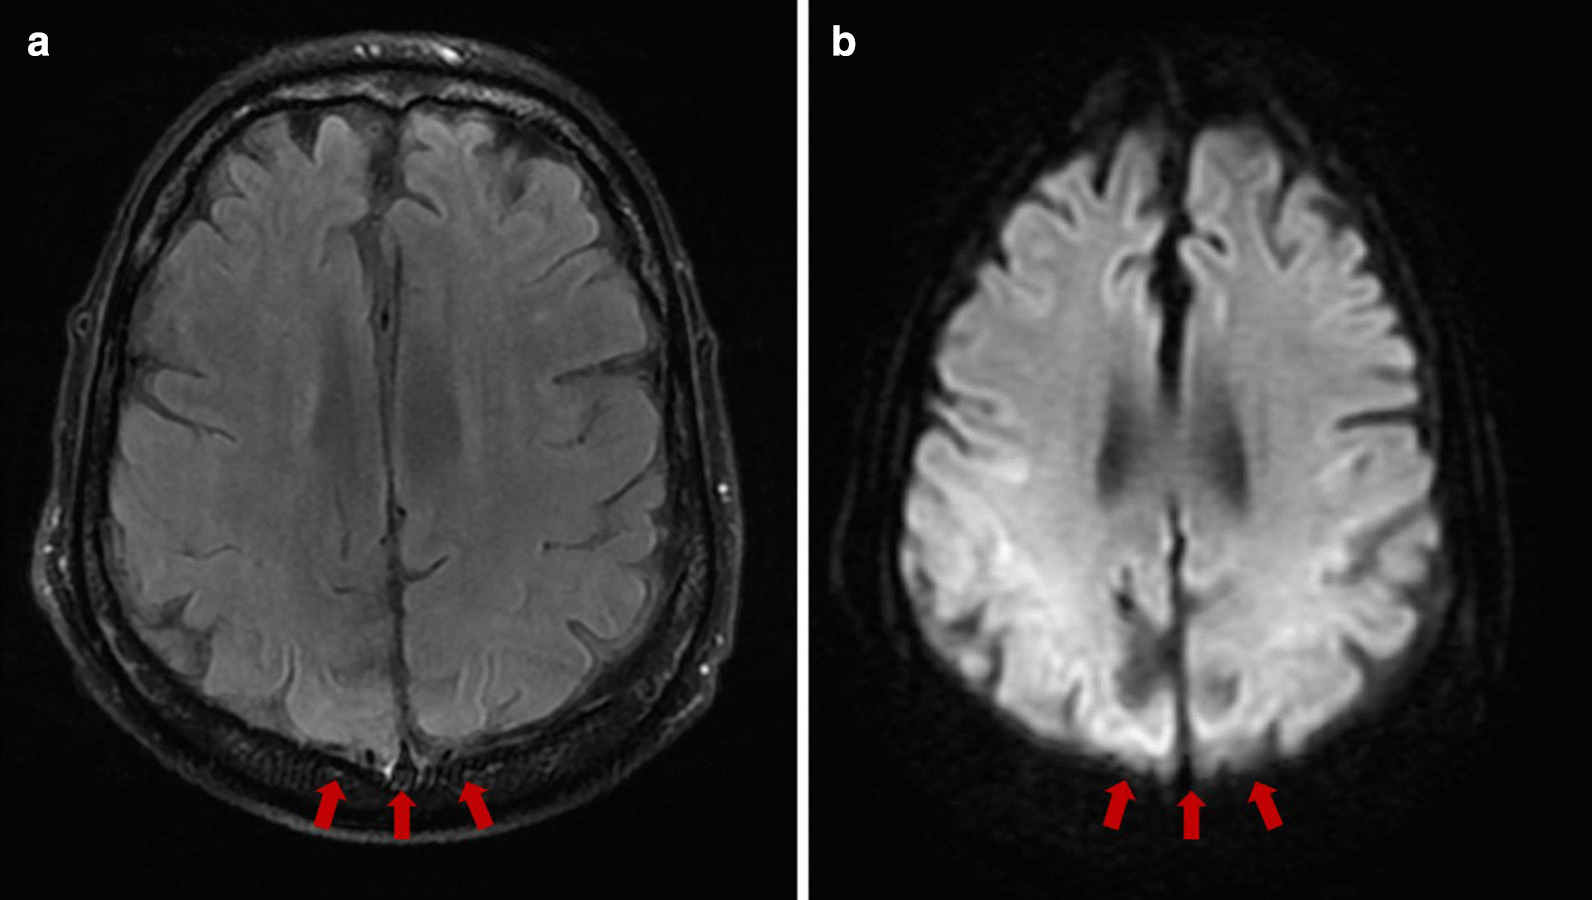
Brain magnetic resonance imaging (MRI). a T2 fluid-attenuated inversion recovery (FLAIR) sequence showed mild hyperintensity of the occipital and inferior temporal lobes bilaterally (red arrows). b Diffusion-weighted imaging demonstrated restricted diffusion in the cortical gray and subcortical white matter of the same regions (red arrows) consistent with edema in the cortical gray and subcortical white matter
